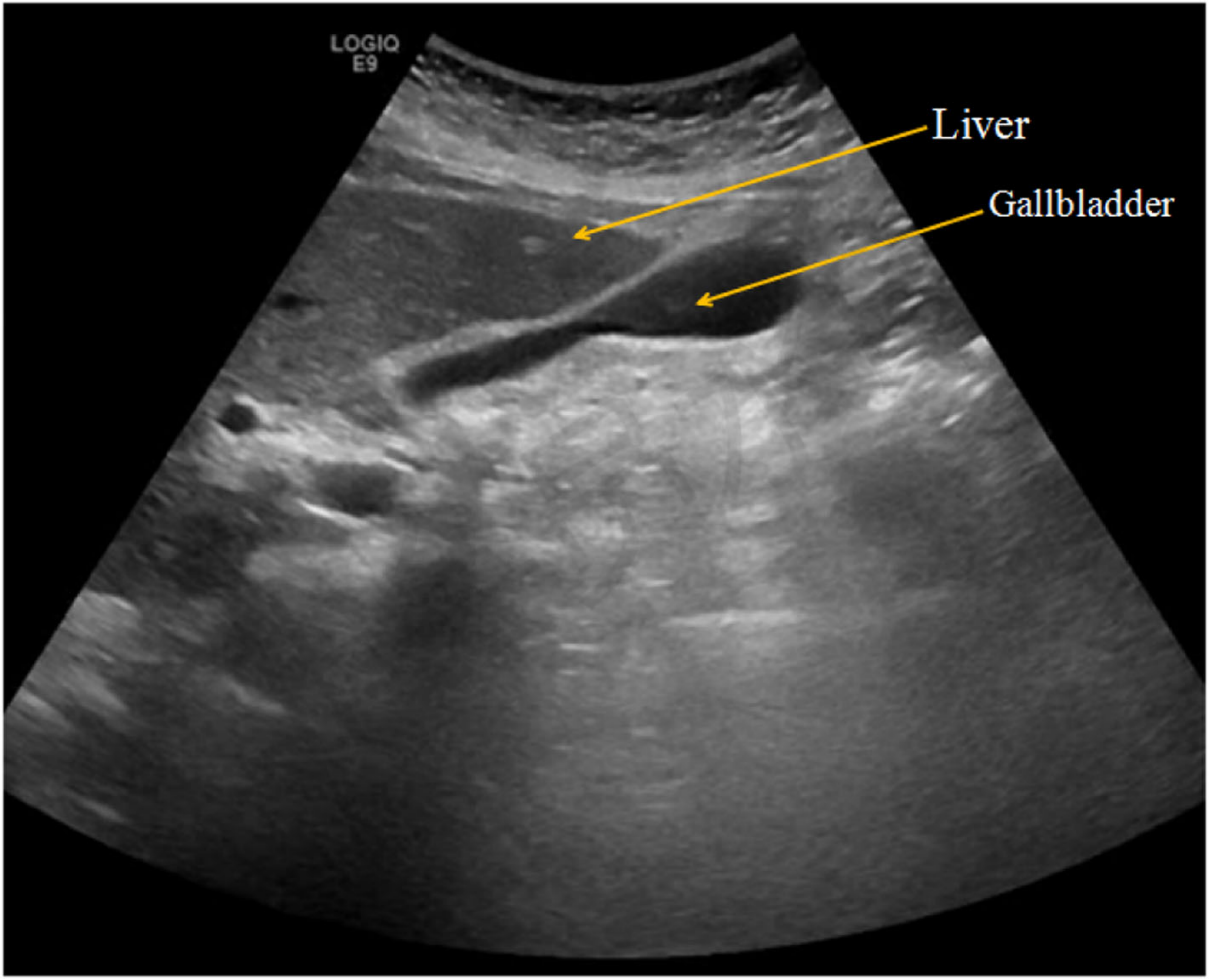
B-ultrasonography examination indicated no obvious abnormalities in the liver, gallbladder, pancreas, spleen, and kidney
